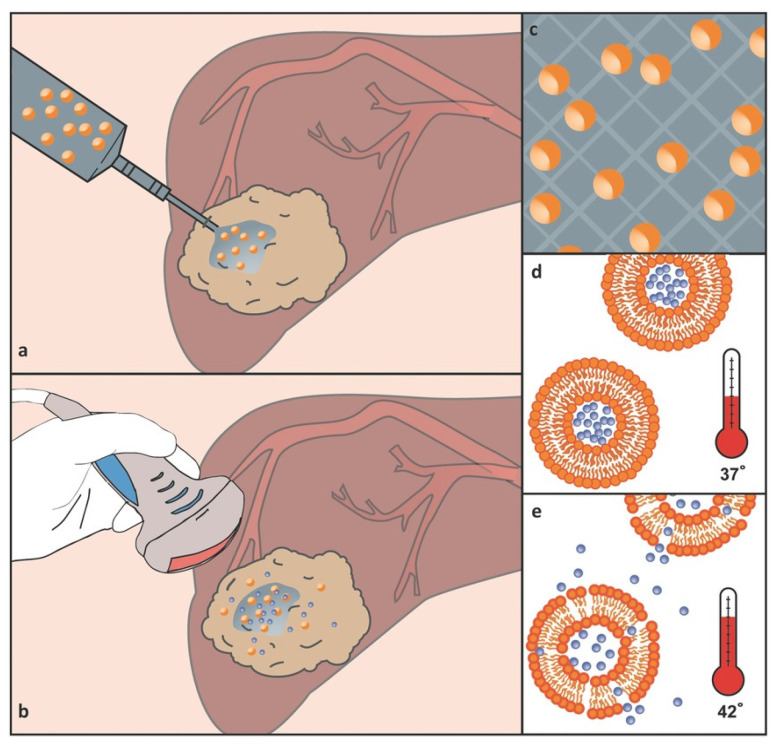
(a) Lipogel is fully injectable, consisting of a CS/β-GP thermo-responsive gel hosting a suspension of Dox-loaded thermo-sensitive liposomes. (b) In situ release from the gel is controlled using minimally invasive hyperthermia, using high-intensity-focused ultrasounds. (c) The majority of liposomes is locked into the gel upon initiation of cross-linking during thermogelation. (d,e) Liposomes sequester the majority of drug at body temperature, but rapidly become more permeable upon mild hyperthermia and release their drug payload. Reprinted from ref. [106]; Copyright 2014 WILEY-VCH.
